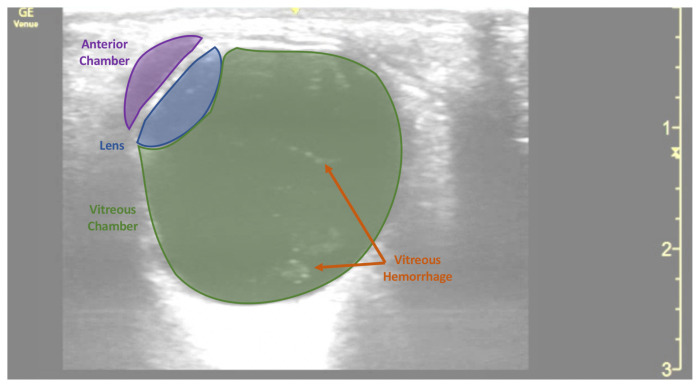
Overgained Ultrasound Video Link: https://youtu.be/bjpWj2b1QjY [Fig f1-jetem-7-3-v20]Ultrasound Video Link: https://youtu.be/LYyN-I0irDs
